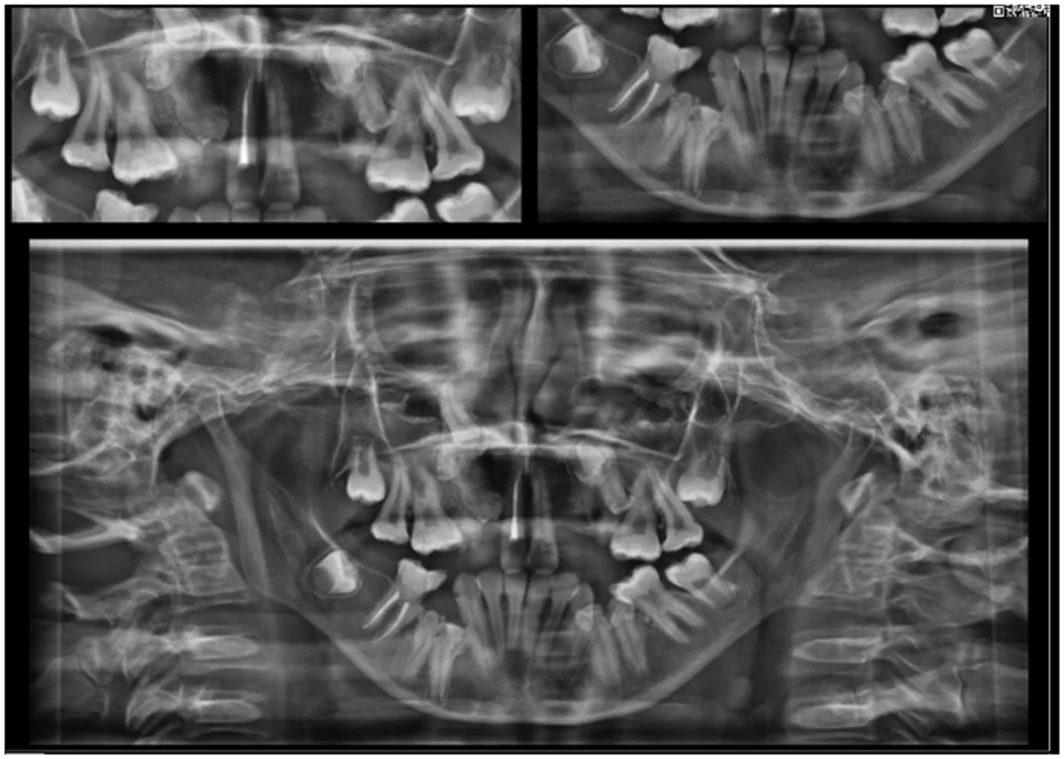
Preoperative panoramic radiograph (OPG) obtained in 2025 (1404 SH) revealing severe clinical deterioration.
